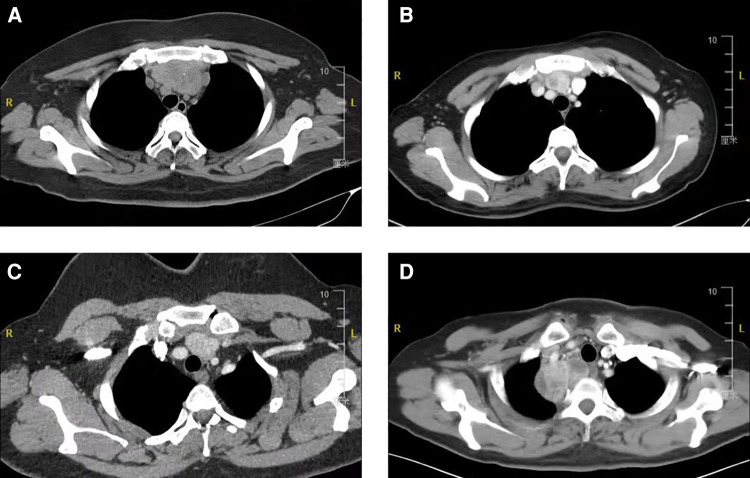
CT images of a retrosternal goiter. A series of patients with retrosternal goiters went through chest CT scan. Masses located in the mediastinum, next to the airway and great vessels, and compression on surrounding blood vessels and trachea could be observed in some cases.
